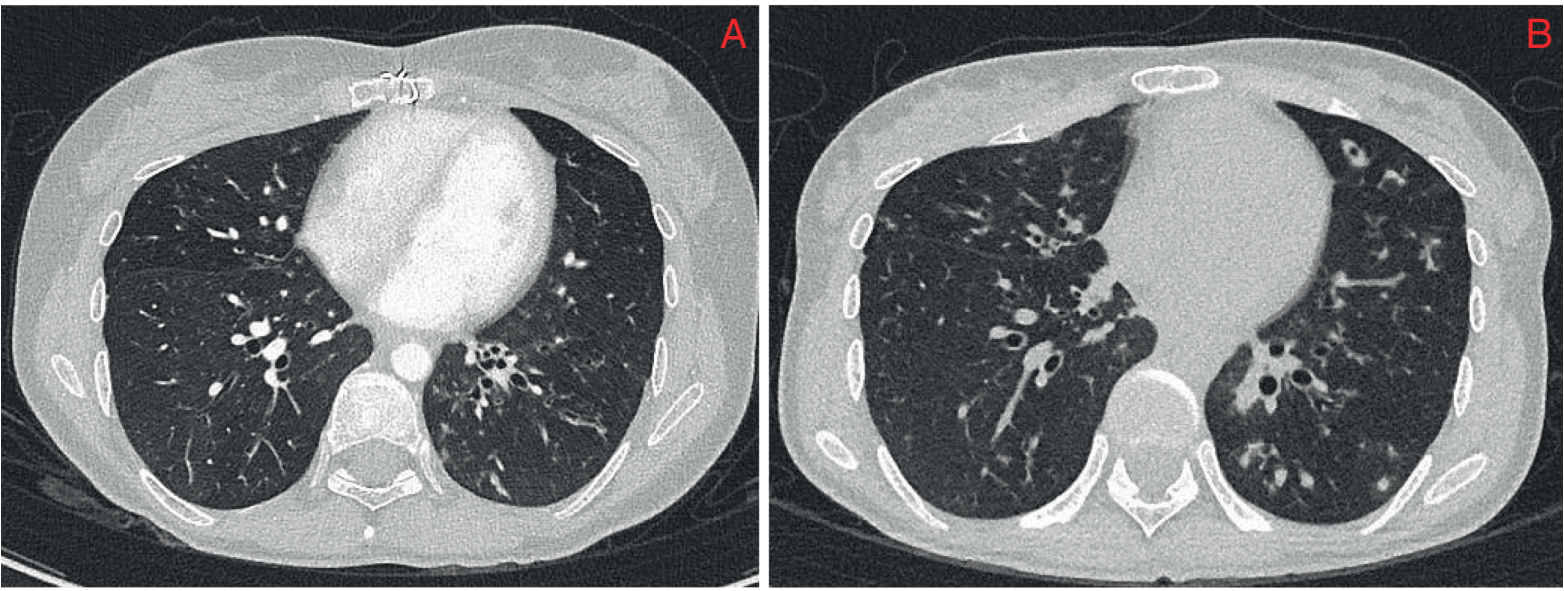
Computed tomography (CT). (A) Chest CT in 2020 showing mild bronchiectasis at the left base. (B) Chest CT in 2022 showing progression of the patient's bronchiectasis with increased nodularity and bronchial wall thickening.
